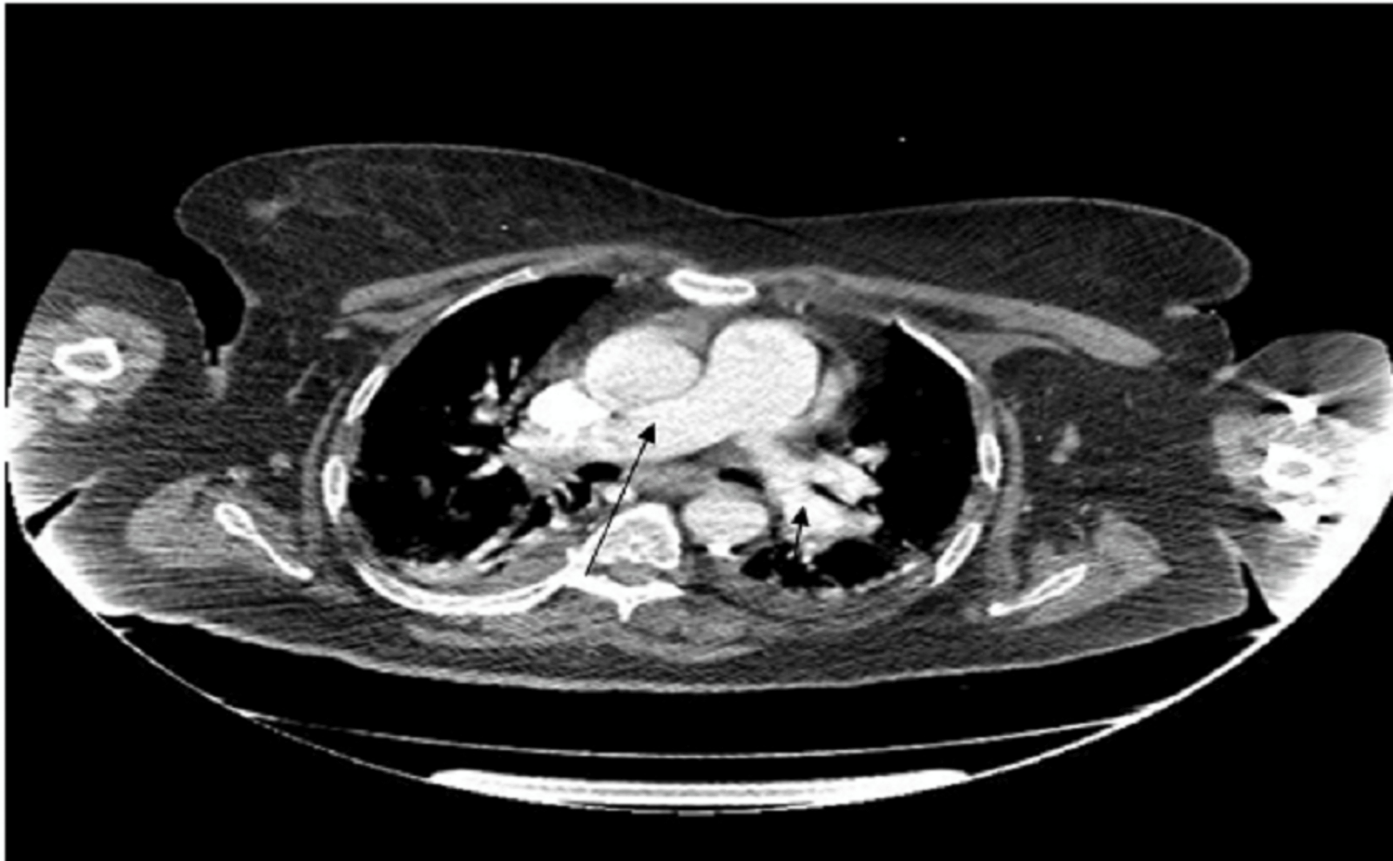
Computed tomography scan of the patient showing a distended gallbladder without pericholecystic edema or biliary dilatation.
